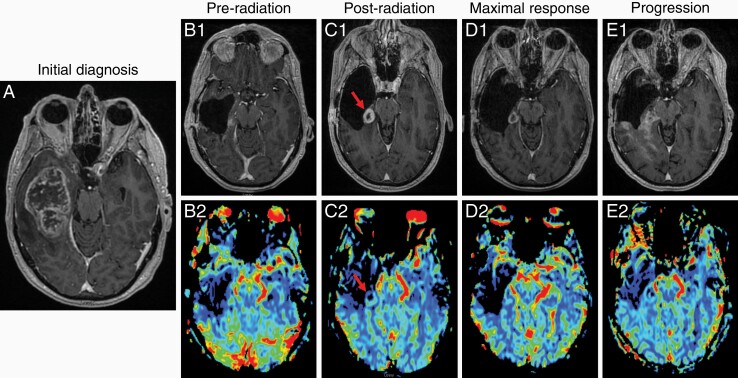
T1-weighted contrasted MRI sequence of the patient’s GBM at the time of diagnosis (A). T1-weighted contrasted sequences (1) and relative cerebral blood volume sequences showing perfusion (2) on MRIs 1 week prior to radiation therapy (B), surveillance 8 weeks after radiation showing disease recurrence (red arrows) with associated perfusion (C), 33 weeks after the initiation of entrectinib where maximal response to therapy was observed (D), and 15 months after entrectinib initiation where disease progression was observed (not all sites of disease pictured). Not pictured here is further rapid, extensive bihemispheric symptomatic progression captured on MRI 15 days after the initial identification of progression. Abbreviations: GBM, glioblastoma; MRI, magnetic resonance imaging.
